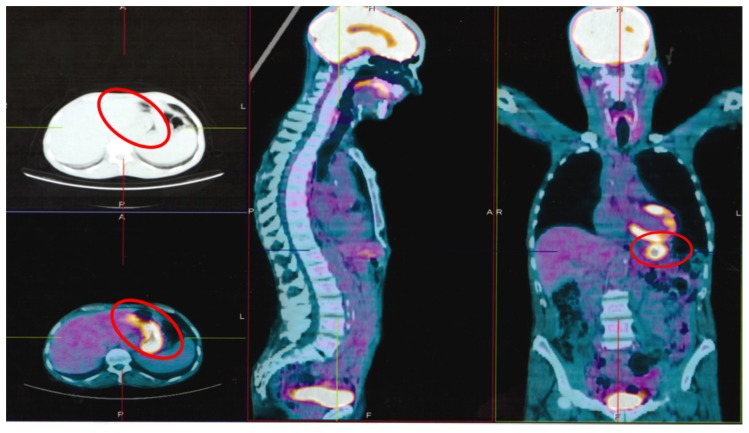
[18F] FDG-PET performed with co-registered CT at the diagnosis (February 2018) show significant areas (indicated in red circles) of increased metabolic activity in abdominal cavity loaded with solid tissue (23 × 14 × 4 cm) which occupies the whole of the mesentery and the retroperitoneal space by displacing the pancreas anteriorly and the intestinal loops laterally (SUV 4.85).
